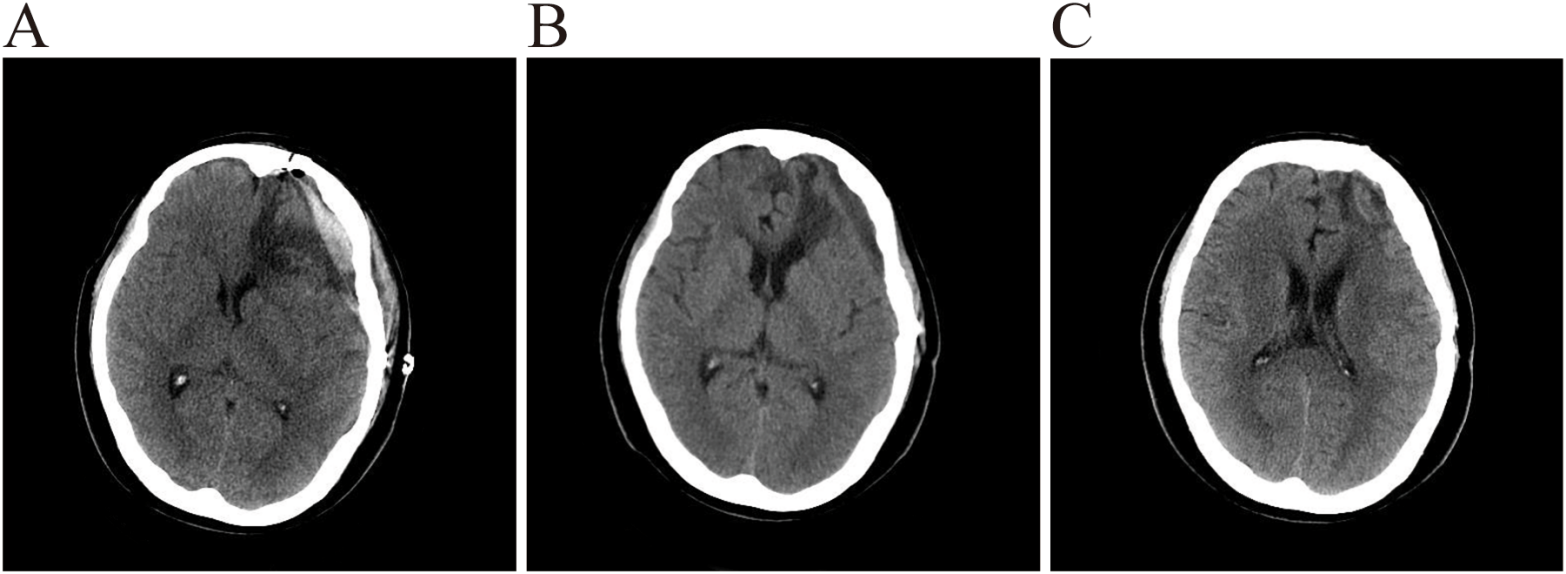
(A) CT demonstrates expected post-surgical changes in the left frontal region. The left frontoparietal calvarium is discontinuous; beneath the inner table, a band-like hyperdensity is seen, maximally ≈1.7 cm thick. Hyperdense streaks are noted within the left frontal lobe parenchyma. A small amount of intracranial pneumocephalus is present. Mild edema of the left frontoparietal brain parenchyma results in compression of the left lateral ventricle and a 0.5 cm rightward midline shift. Subgaleal soft-tissue swelling is evident over the left frontoparietal area. (B) CT again shows post-surgical changes in the left frontal region. The previously hyperdense subdural collection has evolved into a hypodense band, now ≤1.0 cm thick. Midline shift has decreased to ≈0.3 cm rightward. Subgaleal soft-tissue swelling is markedly reduced. (C) CT demonstrates persistent post-surgical changes. The left frontoparietal bone remains discontinuous. A thin, hypodense extra-axial collection persists beneath the inner table, measuring ≤0.4 cm at its thickest point. No significant mass effect is present; the left lateral ventricle is well visualized, and midline structures are essentially centered.
